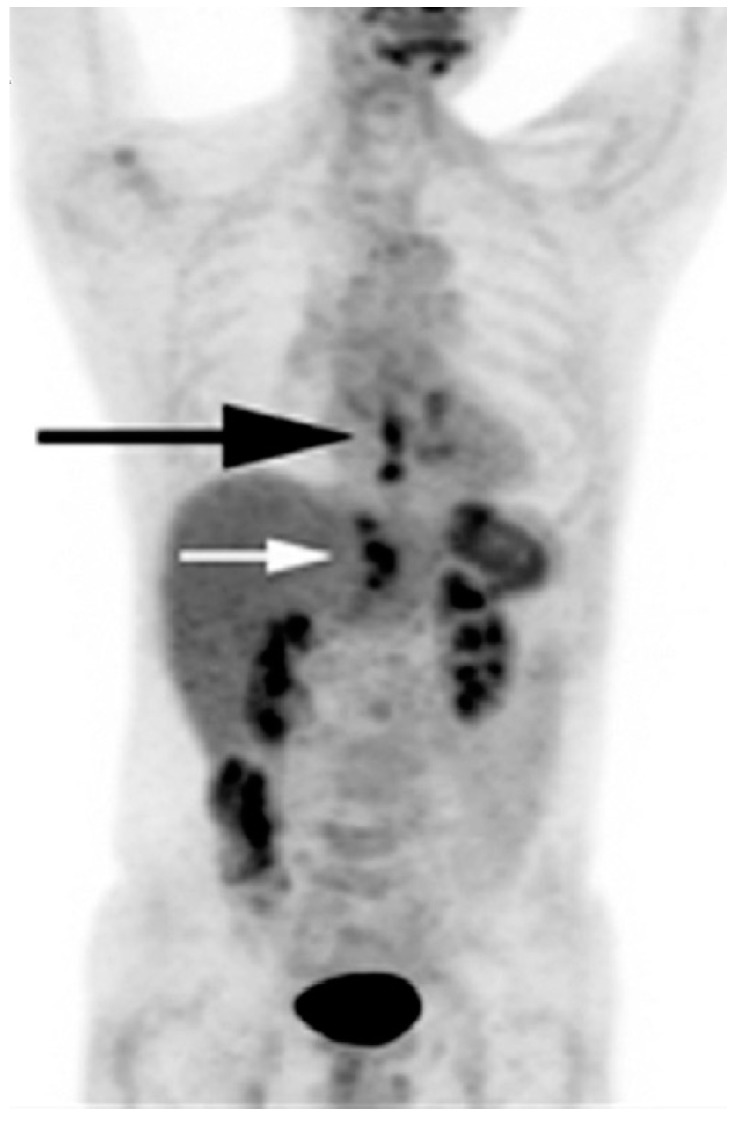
Hepatic radiation injury in a patient who underwent radiotherapy for distal esophageal cancer. The coronal maximum projection image (FDG PET image) shows residual esophageal tumor hypermetabolism (SUVmax = 3.4) (black arrow) and hypermetabolic areas (SUVmax = 3.5) in liver segments I, II, and III (white arrow). (Used with permission from [61]). SUV, standardized uptake value.
